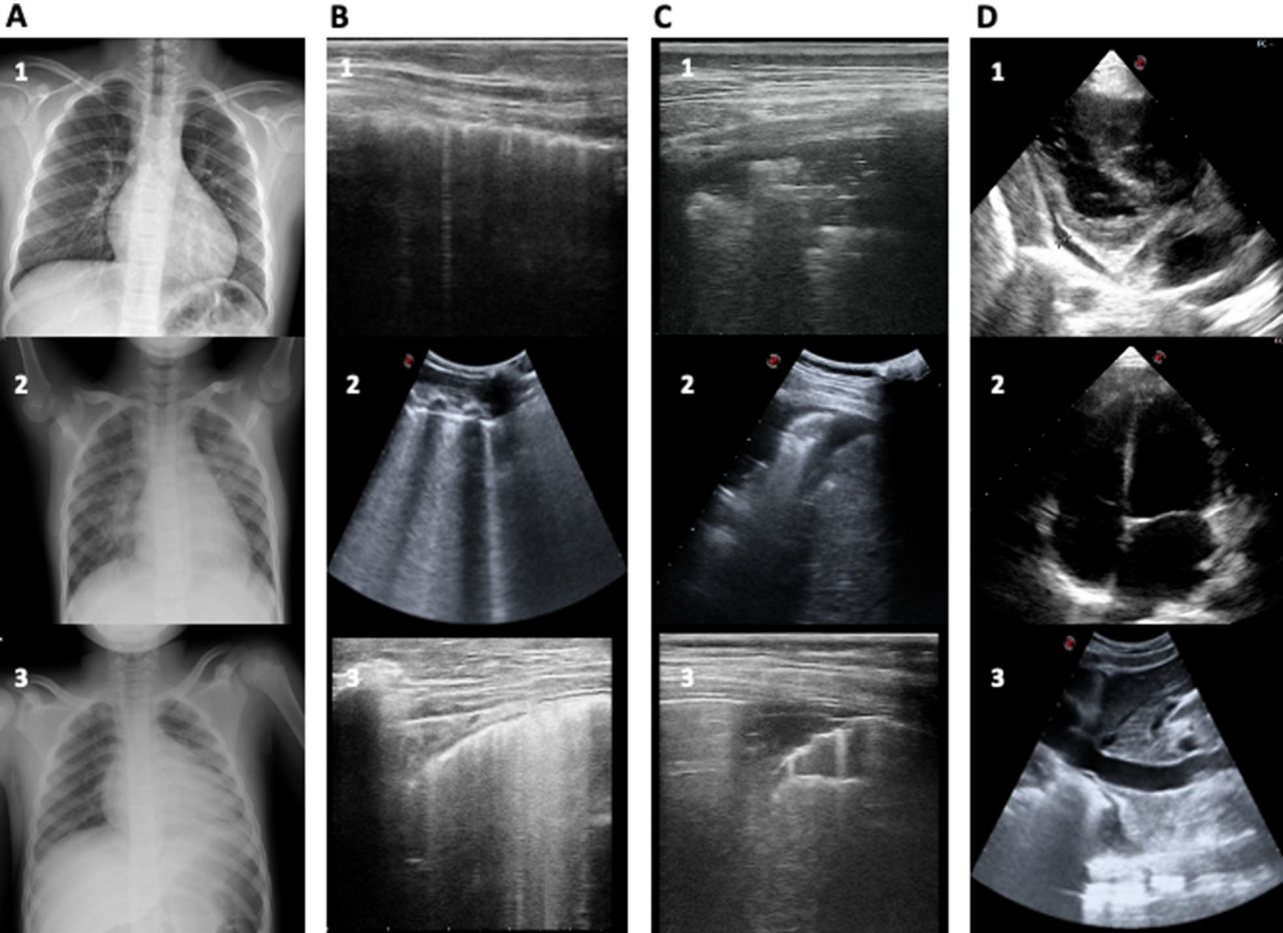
Imaging findings: A chest X-ray findings; B LUS exam: B1 initial interstitial pattern, B2 vertical artifacts, B3 white lung; C LUS exam: C1 lung consolidations; C2, C3 lung consolidations with pleural effusion; D RUSH exam: D1 pericardial effusion, D2 dilated cardiac chambers, D3 plethoric IVC
